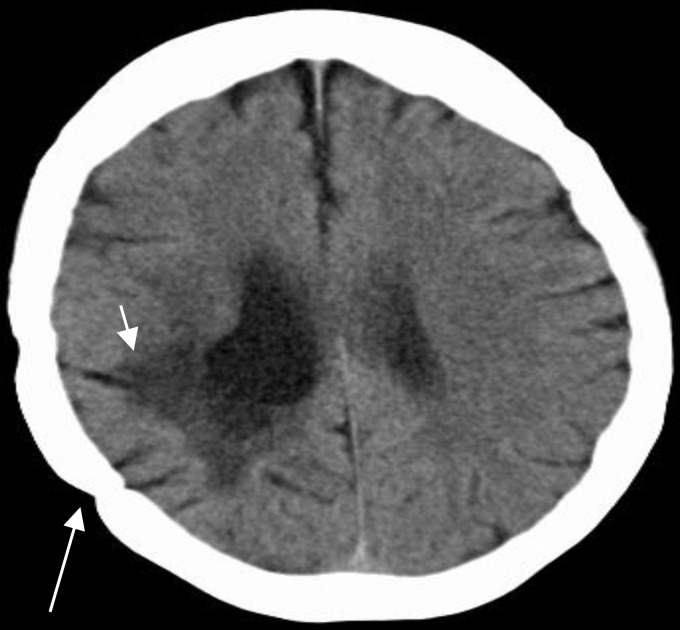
Noncontrast brain computed tomography (CT) demonstrating previous right parietotemporal craniotomy (long arrow) and the underlying encephalomalacia (short arrow)
